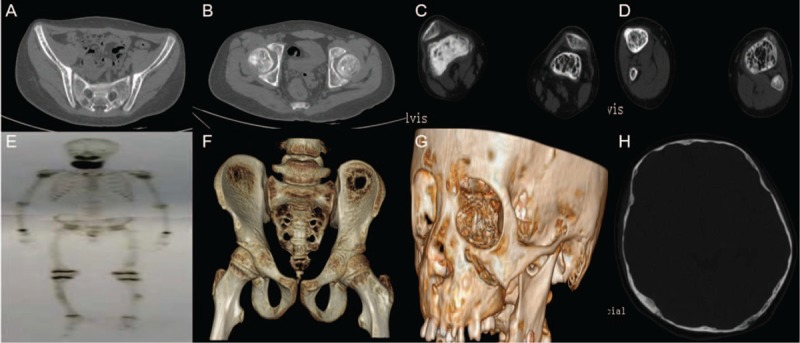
(A & B) Axial computed tomography (CT) scan revealed that coccygeal vertebra, ilium and femur neck were all undergoing osteopenic changes. (C & D) Radiolucent images, with foamy trabeculae and thinner cortex of femur, patella, tibia, and fibula. (E) Technetium scintigraphy showed increased tracer uptaker in the chin area. (F) Two bony defects were identified in reconstructed pelvic CT images. (G & H) CT showed loss of lamina dura, decreased skull bone density, and sporadic bony defects.
